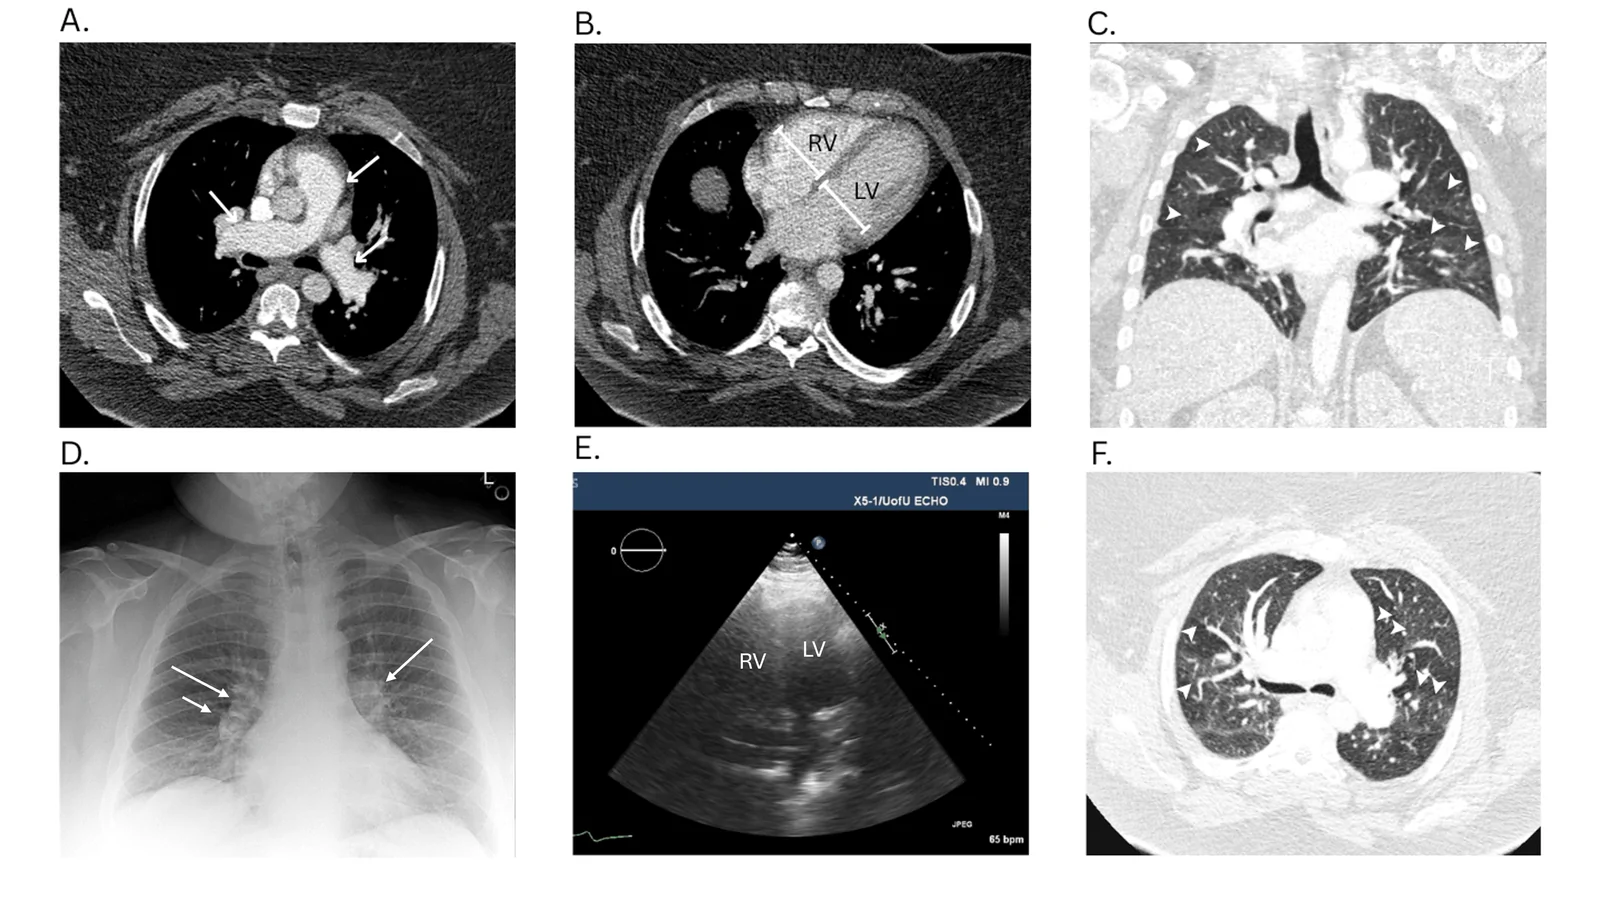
Imaging findings in Case 1. Axial CT images show enlargement of the central pulmonary arteries (A, arrows) and right ventricular (RV) enlargement (B). Coronal and axial CT images displayed in lung windows (C and F) demonstrate moderate, diffuse, patchy mosaic attenuation (arrowheads) and relatively small peripheral pulmonary arteries. A frontal chest radiograph (D) shows enlarged branch pulmonary and interlobar arteries with attenuated peripheral pulmonary vasculature. An apical four-chamber transthoracic echocardiographic image (E) provides limited visualization of the right heart and left atrium.
